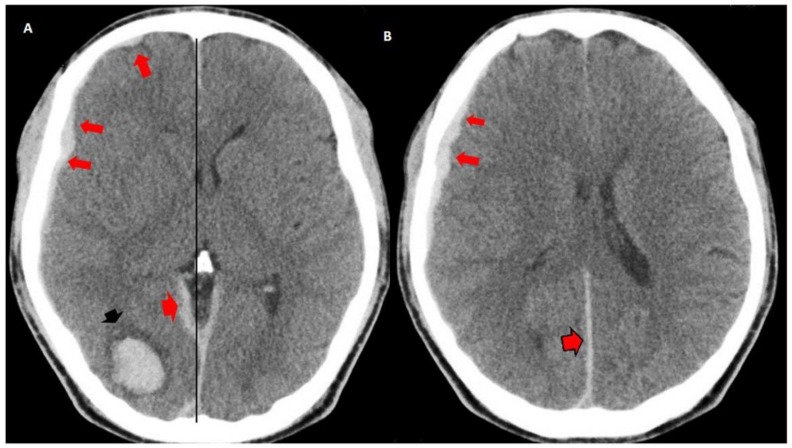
Simple brain computed tomography (CT): intraparenchymal hematoma in the right occipital region (black arrow head in (A)). Right frontal laminar subdural hematomas: (red arrows in (A,B), subdural hematoma at the tent of the cerebellum (red arrow head) and posterior interhemispheric subdural hematoma in contact with the sickle (red arrow head with black border in (B)). Mild mass effect on the ipsilateral ventricle, effacement of the subarachnoid space. Midline displaced 0.5 cm.
